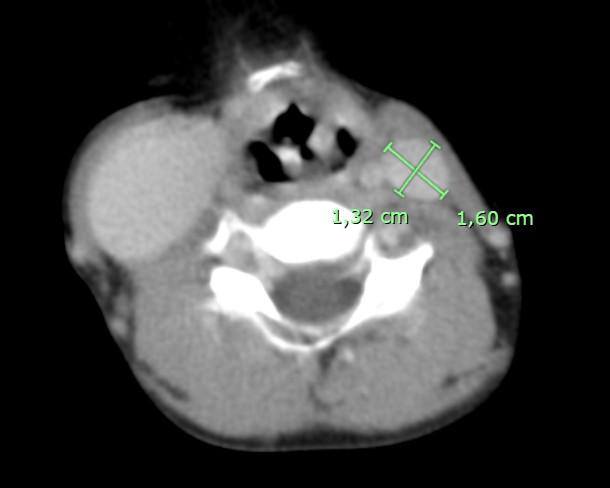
CT image in axial plane with intravenous contrast, showing the left internal jugular vein.
